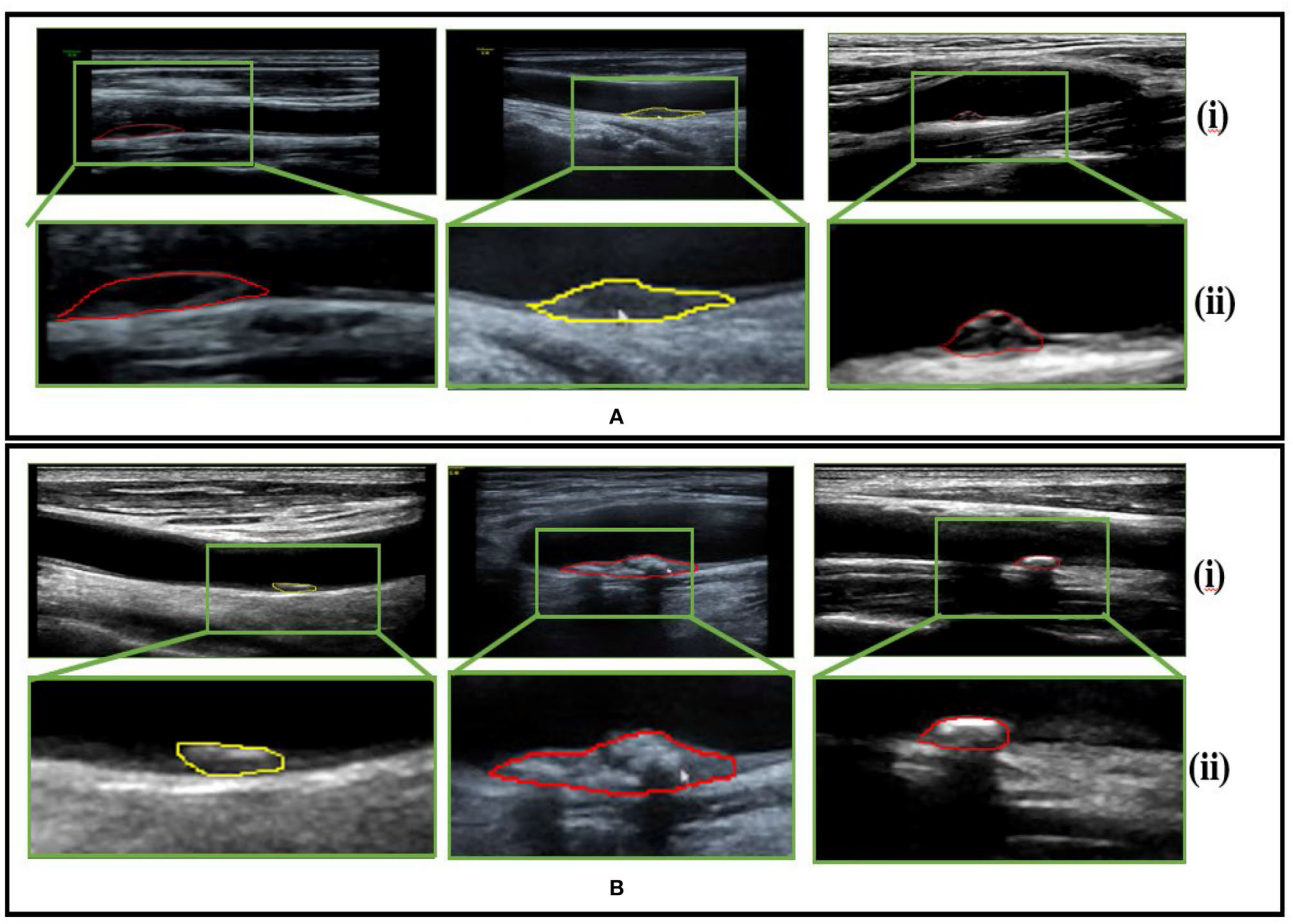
Sample dataset (A) pertains to vulnerable carotid plaque ultrasounds images scans and (B) belongs to stable carotid plaque ultrasounds images scans, whereby (i) and (ii) represent the color Doppler image scans and their respective enlarged views for clarity.
